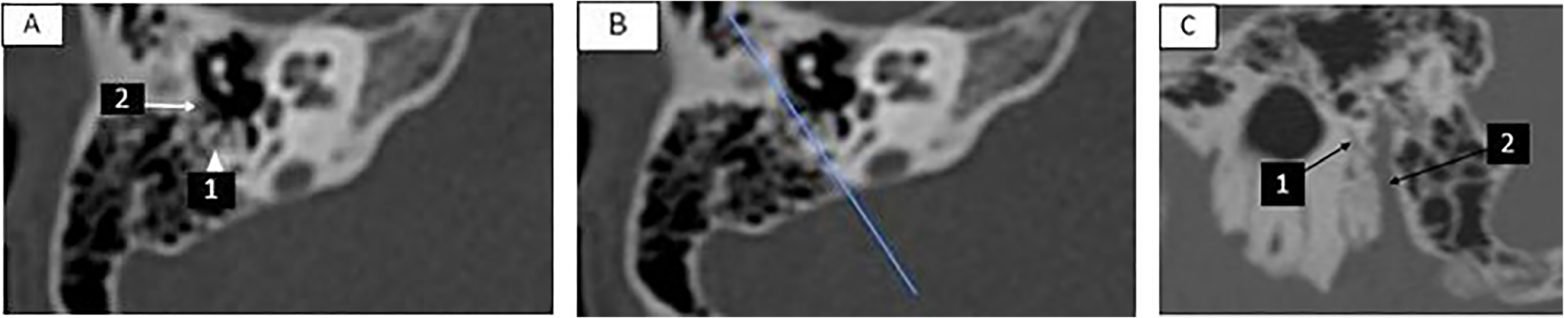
The radiological maneuver of obtaining the chorda-facial angle; A right axial cut of the HRCT. (1 = mastoid portion of the facial nerve, 2 = chorda tympani nerve). B Blue line joining the mastoid portion of the facial nerve and the chorda tympani nerve; representing the plane of reconstruction for transverse oblique short axis (parasagittal) view. C Oblique transverse oblique cut at the plane of the MPFN-CTN line. (1 = chorda tympani nerve, 2 = mastoid portion of the facial nerve)
